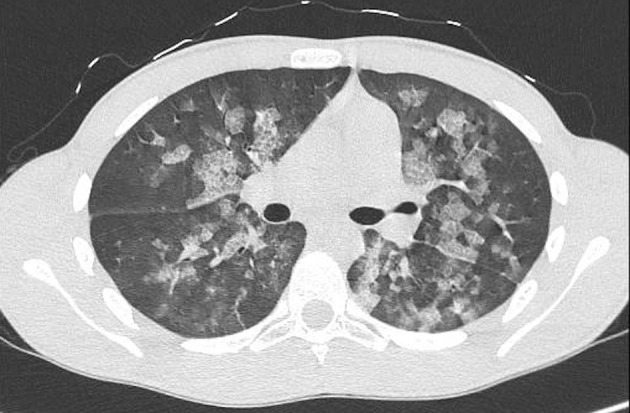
Chest CT scan without contrast medium confirming the diagnosis of DAH.
